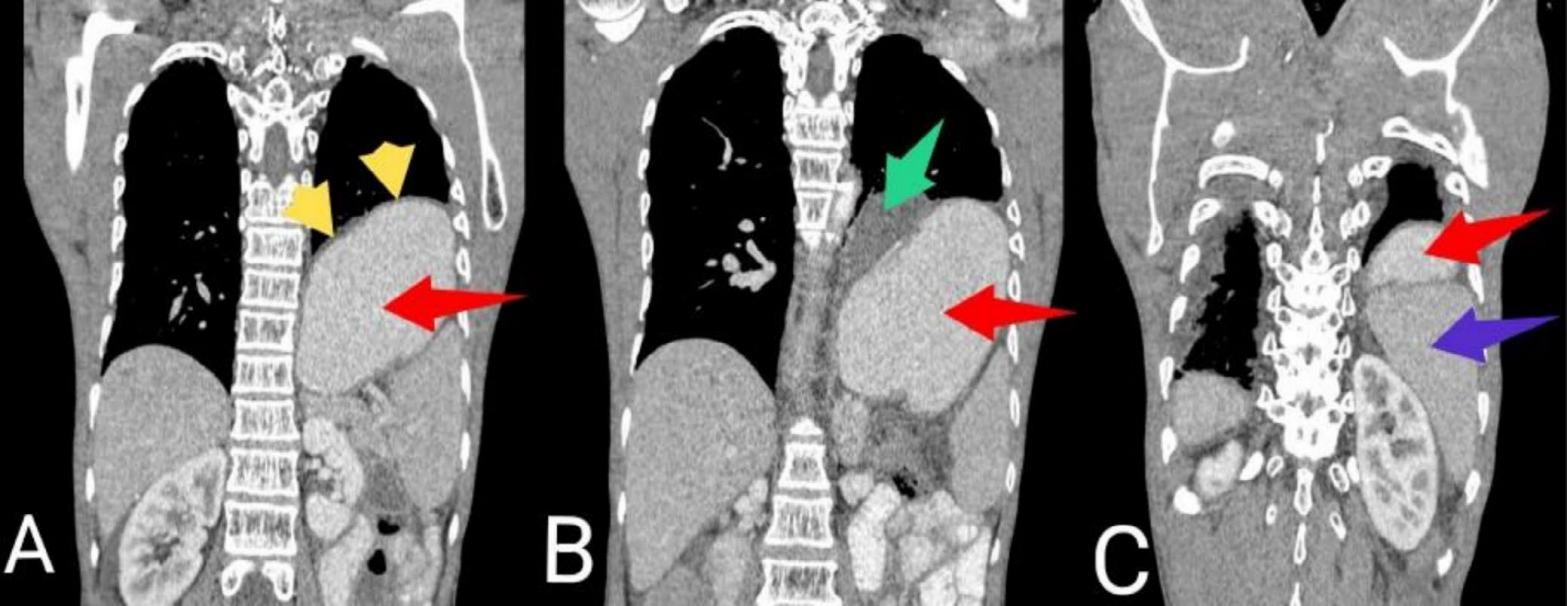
Contrast‐enhanced coronal chest CT scan images in mediastinal window showing significantly elevated intact smooth‐contoured left hemidiaphragm (yellow arrows in A) with upward displacement of the stomach (red arrows in A, B and C), mesenteric fat (green arrow in B) and spleen (blue arrow in C) into the left hemithorax.
